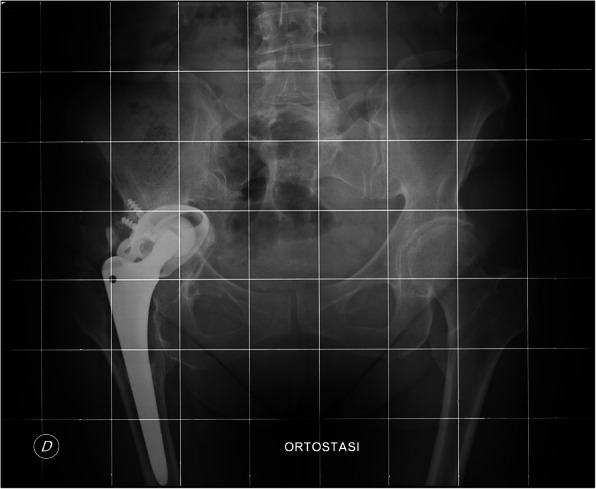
X-ray of aseptic loosening of right hip of 54 years old female
